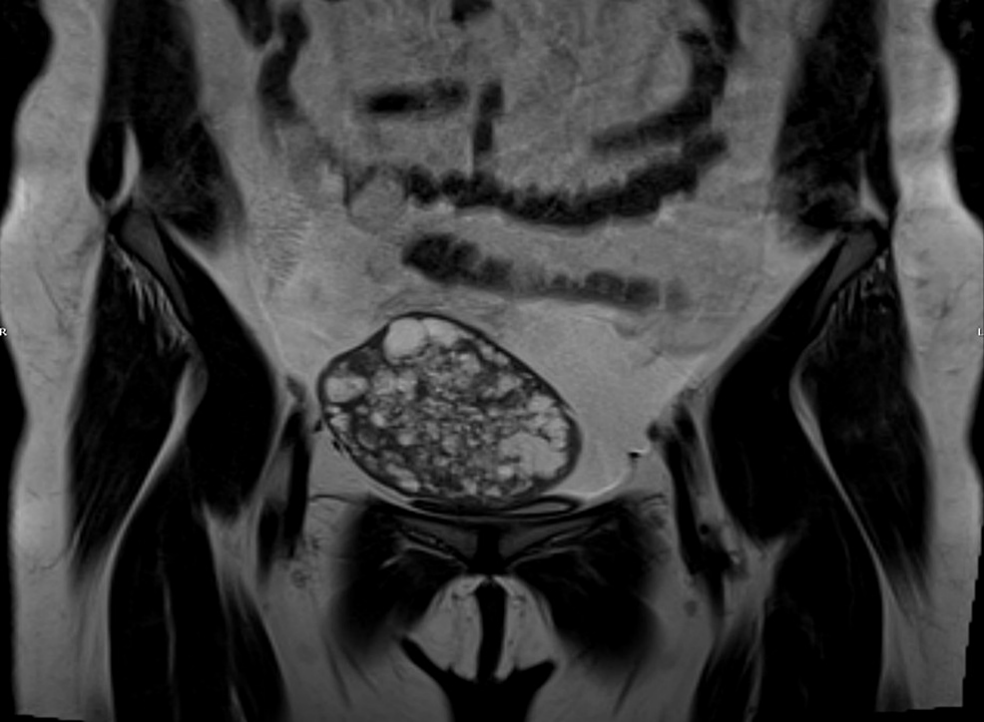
Coronal pre-operative MRI of pelvis done in 2012 shows a complex cystic mass lesion in the pelvis – primary granulosa cell tumor of the ovary
